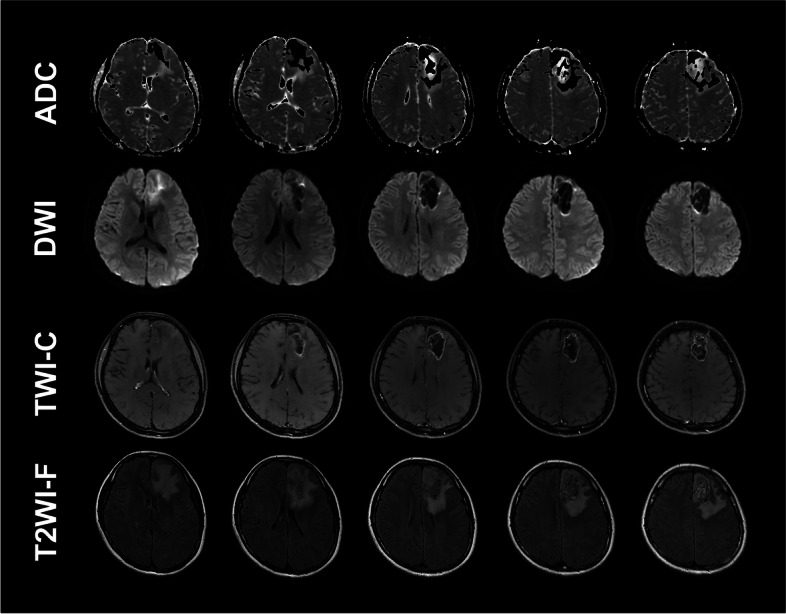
Brain MR images of the patients with grade IV glioma, eight months after the ^125^I implantation. ADC, DWI, T1WI-contrast, and T2WI-flair images showed the glioma shrink obviously after the ^125^I implantation. The brain edema also improved compared with the brain images eight months ago
